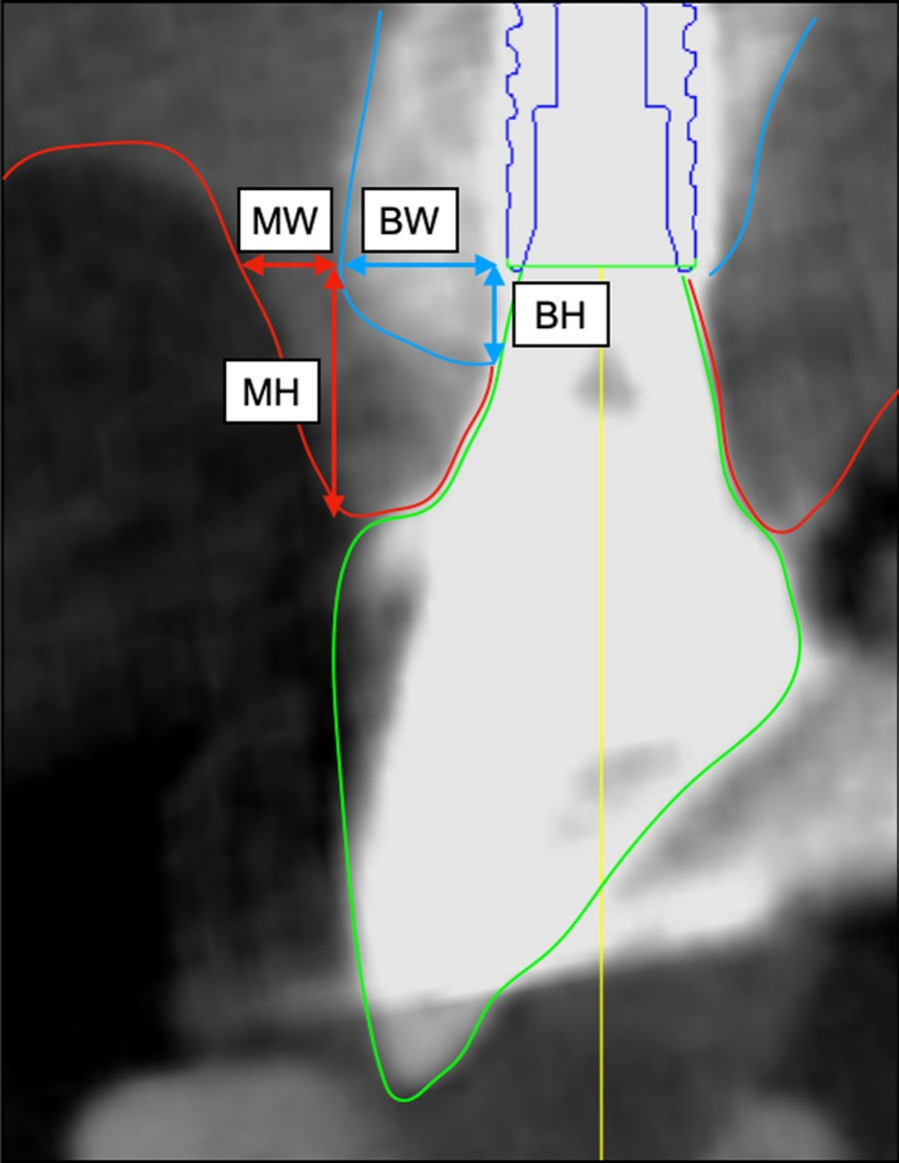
Representative images of examined implants with CBCT scans. Hard tissue width and soft tissue width of labial/buccal aspect on implant platform was measured as BW and MW. Hard tissue height and soft tissue height on labial/buccal aspect of implants were also measured as BH and MH. *BW* bone width, *MW* mucosa width, *BH* bone height, *MH* mucosa height
